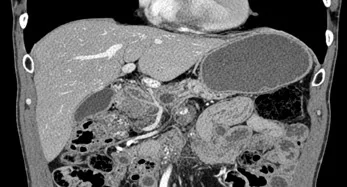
Computed tomography showing findings consistent with chronic pancreatitis. Source: Personal archive.
